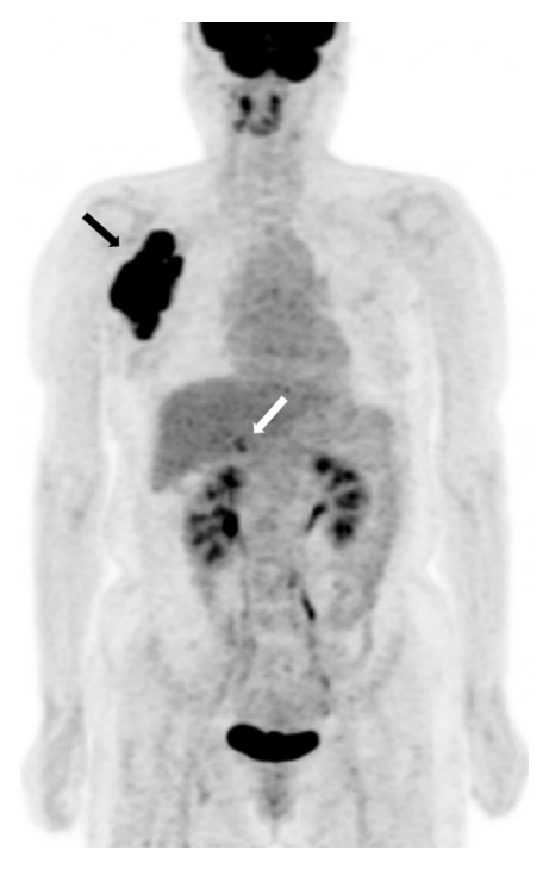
^18^F-FDG-PET scan shows two focal FDG-avid uptakes in the right axilla (SUV max 12.7, black arrow) and right adrenal gland (SUV max 4.7, white arrow). SUV max: maximum standardized uptake value. ^18^F-FDG-PET: ^18^F-fluorodeoxyglucose-positron emission tomography.
